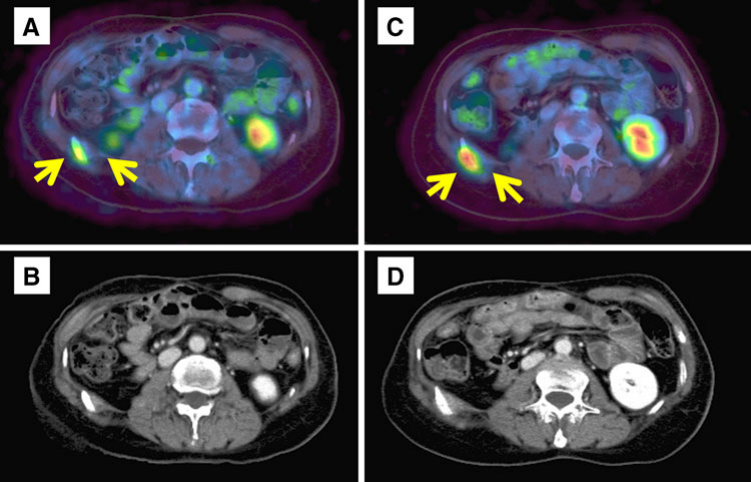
Progression of the tumor on PET and CT. PET and CT show a gradual increase in tumor size. a, b September 2008. c, d April 2009
